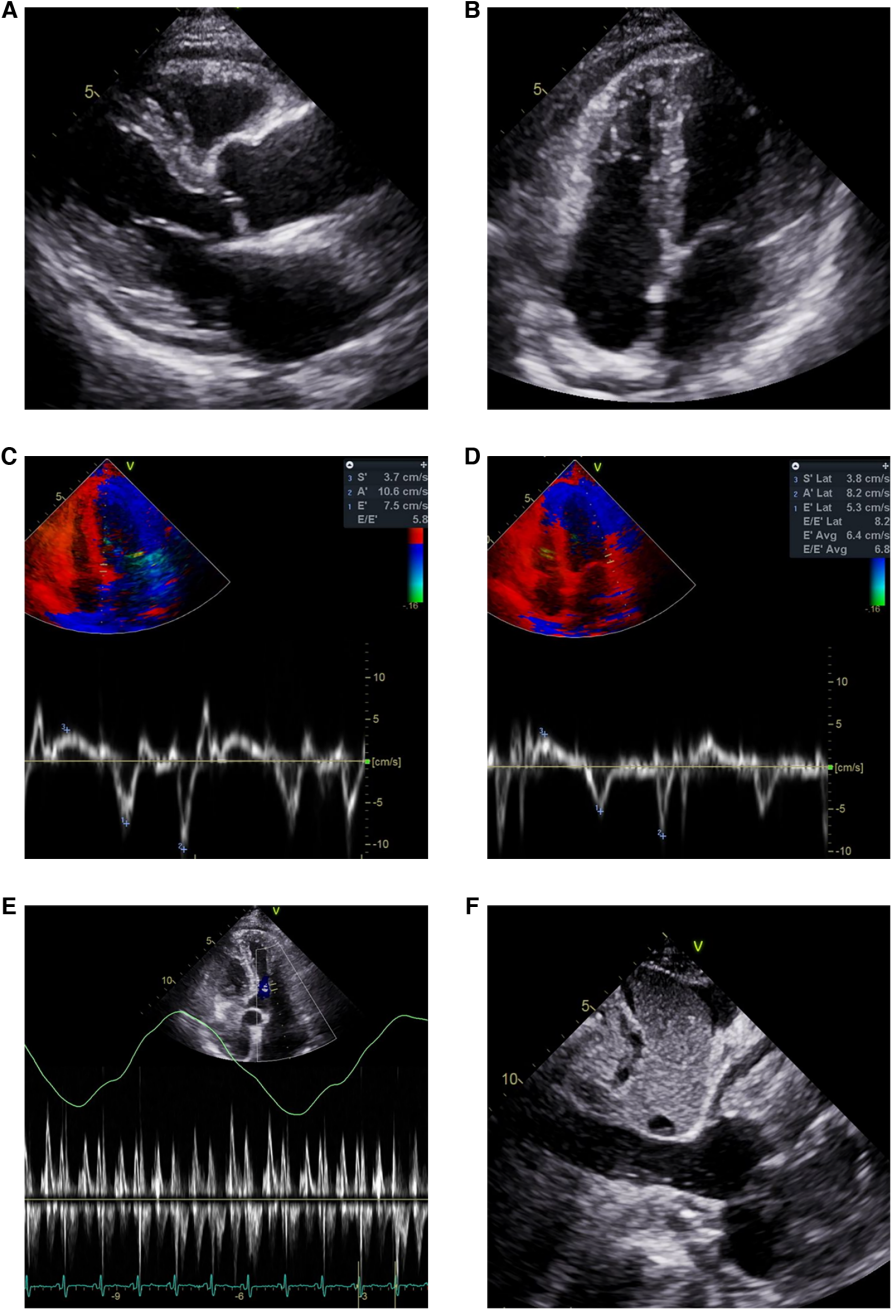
Echocardiogram showing pericardial effusion and constrictive physiology. Small amount of pericardial effusion and thickened pericardium in (A) parasternal long axis view and (B) modified 4-chamber view. Annulus inversus findings showing (D) decreased lateral e’ velocity compared to (C) septal e’ velocity. (E) Mitral inflow showing significant variations during respiration (F) Plethoric inferior vena cava during inspiration.
